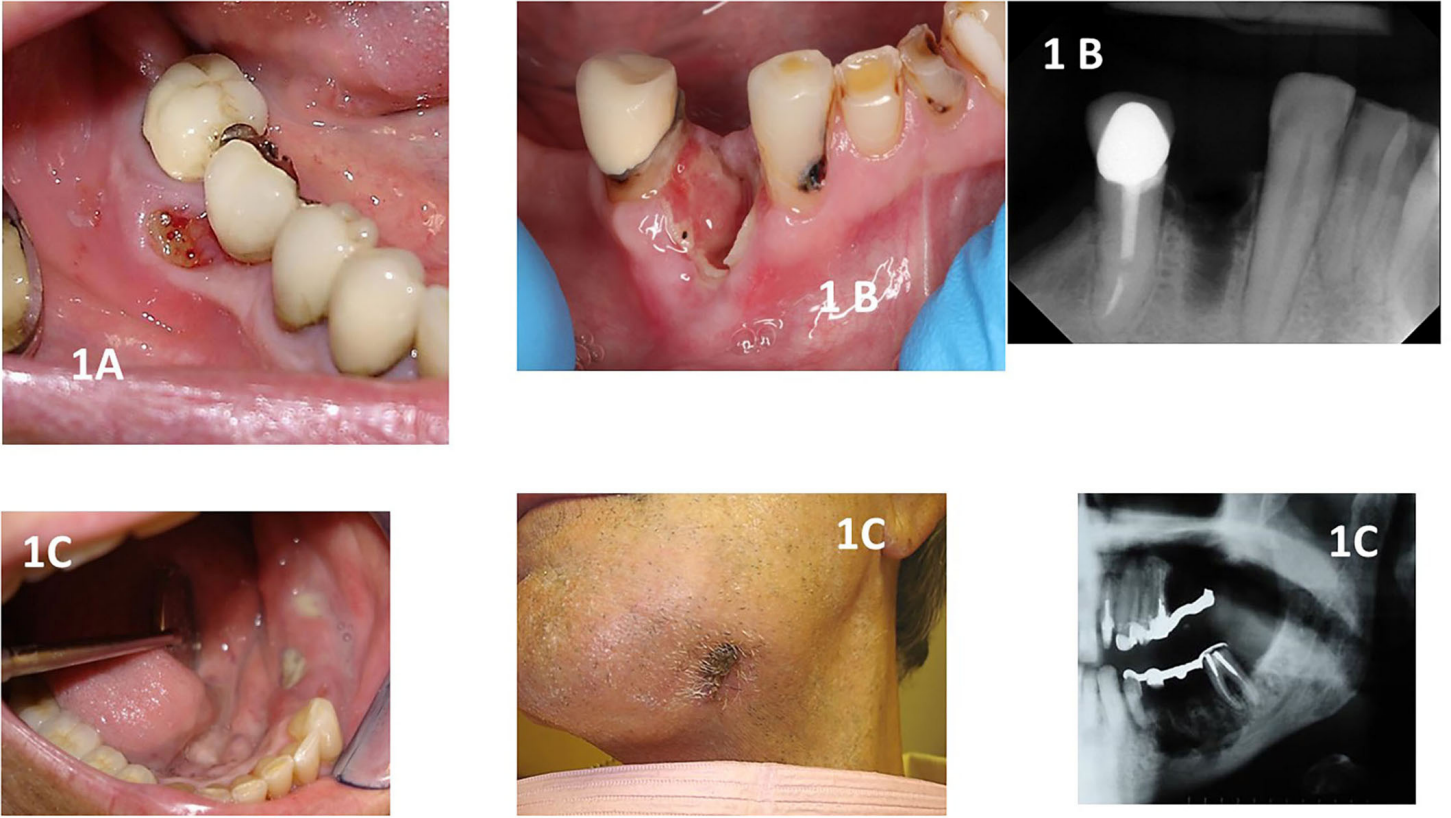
Shows clinical and radiographical images of different stages of MRONJ. (A): stage 1 showing a patient with breast cancer taking zoledronic acid with a small asymptomatic area of exposed necrotic bone that was affecting the use of a removable prosthodontic appliance. (B): shows a patient taking denosumab who had a recent dental extraction. The site was not healing, and exposed alveolar bone could be seen. The patient was in pain and did not respond to antibiotics. The radiographical image shows the non-healing alveolus. (C): shows a patient with multiple myeloma with Stage 3 MRONJ. The patient was in pain and presented to the clinic with swelling of the alveolar mucosa and several areas of infected and exposed bone with pus drainage. The patient complained of paresthesia in the area and had a strong mal odor. One can observe an extraoral fistula on the left submandibular area. The radiograph shows the extensive area of bone destruction, placing the patient at risk for a pathological fracture. Note that the fixed prosthodontic appliance was removed, revealing the exposed bone seen in this figure.
